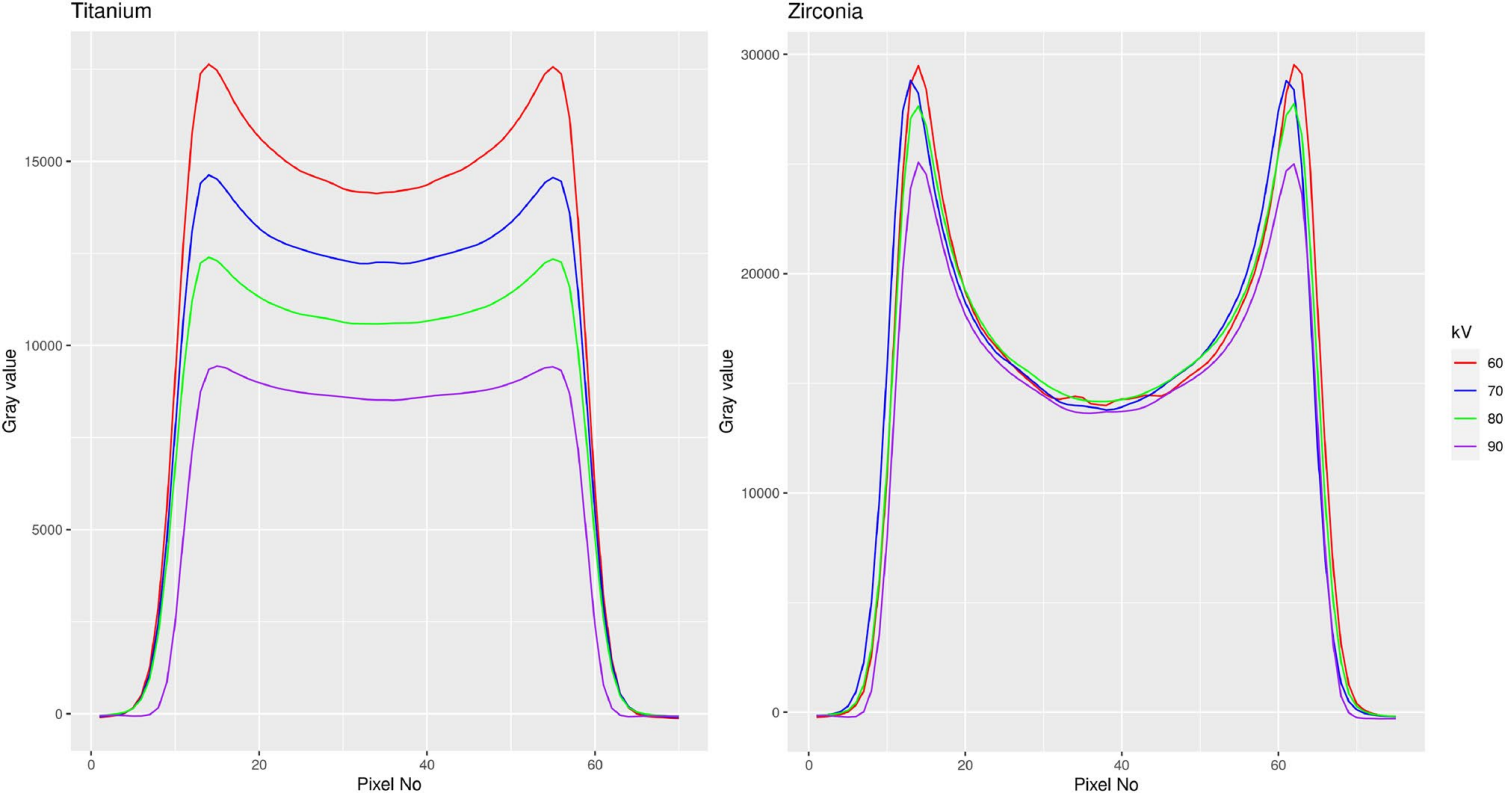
Numerical comparison of the actually occurring beam hardening in the reconstructed 3D CBCT-images of the implant samples. The plot shows averaged gray value-profiles through the implant cross-section (see Fig.5). Note the significant beam hardening artefact for Y-TZP equal for all four energies and representing as a significant reduction in gray value in the center of the implant image of approximately 50%.
